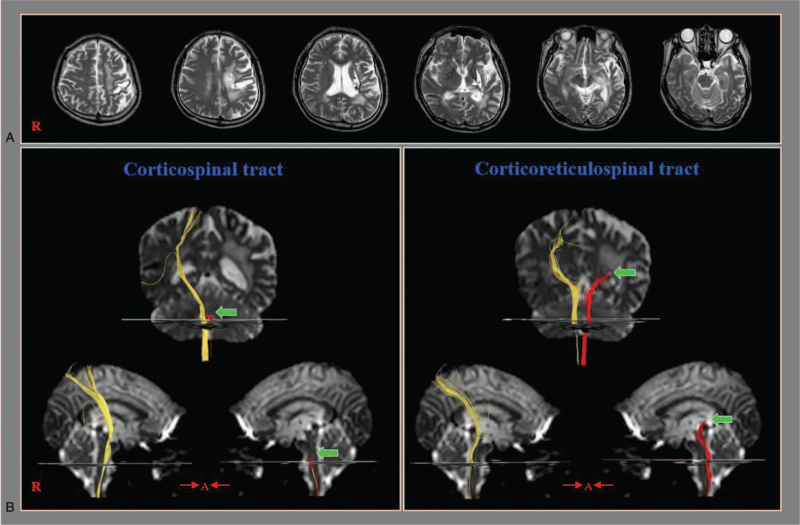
(A) T2-weighted brain MR images at 19 months after onset showing leukomalactic lesions in the fronto-parieto-temporal lobes and basal ganglia. (B) The left corticospinal tract and cortico-reticular-spinal tract show the discontinuations at the brainstem and subcortical white matter, respectively (arrows). In contrast, the right corticospinal tract and cortico-reticular-spinal tract reveal intact integrities.
